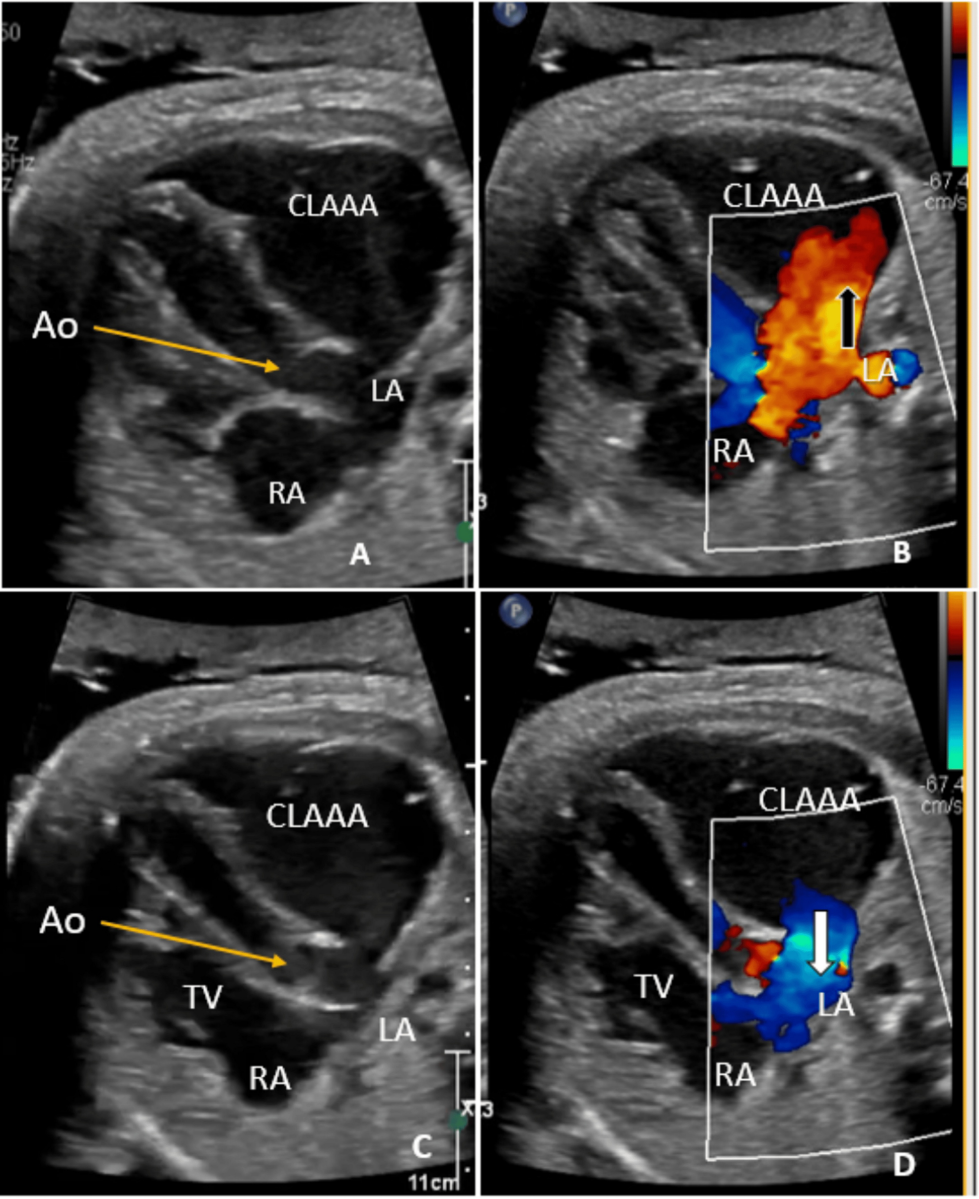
(A-D) Fetal echocardiography in a long-axis view, tilted cranially from the four-chamber view, showed a single cystic mass adjacent to the left side of the heart representing the CLAAA. Color Doppler demonstrated flow from the CLAAA to the LA (bold white arrow, image D) during diastole when the TV is open, and flow returning to it (bold black arrow, image B) during systole when the TV is closed, with forward flow seen across the Ao. The slim orange arrow indicates the level of the Ao. Ao: aortic valve; CLAAA: congenital left atrial appendage aneurysm; LA: left atrium; RA: right atrium; TV: tricuspid valve
